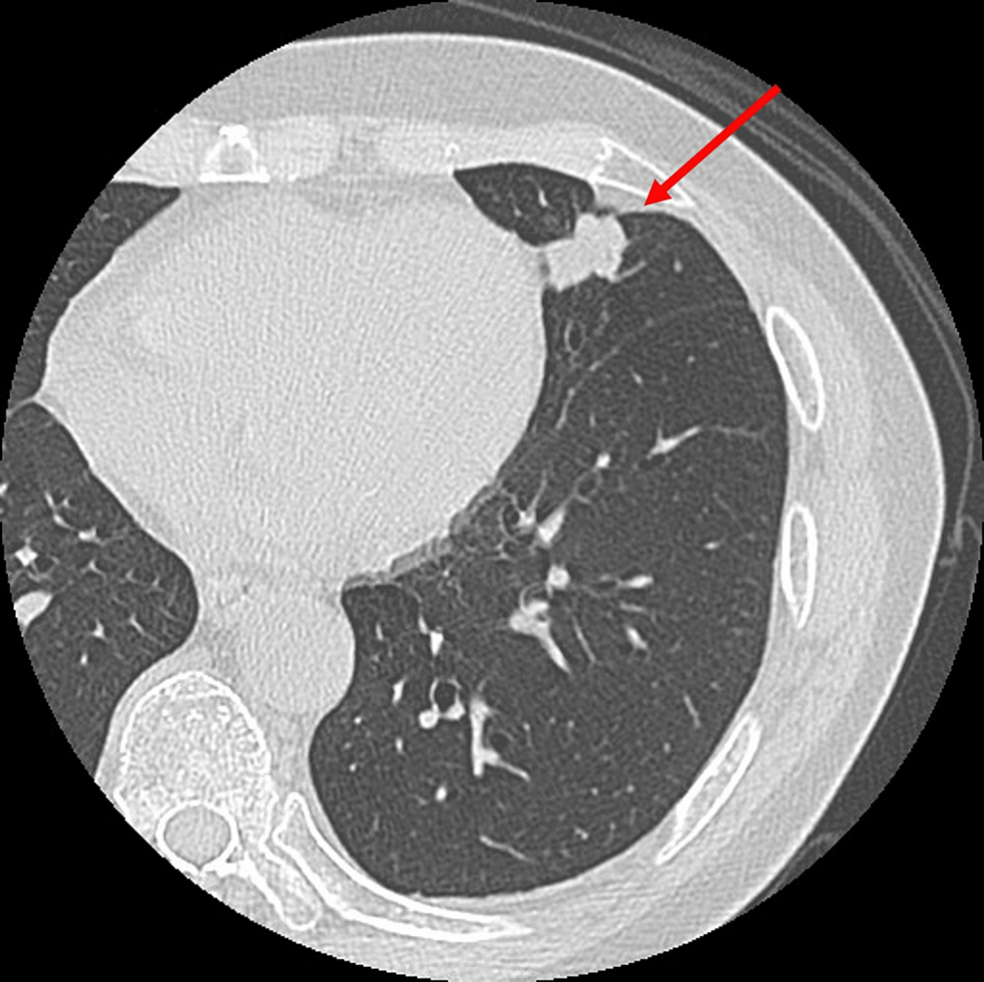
Chest CT A chest CT showed a tumor in the lingual region of the left lung with a long diameter of 20 mm (red arrow).
